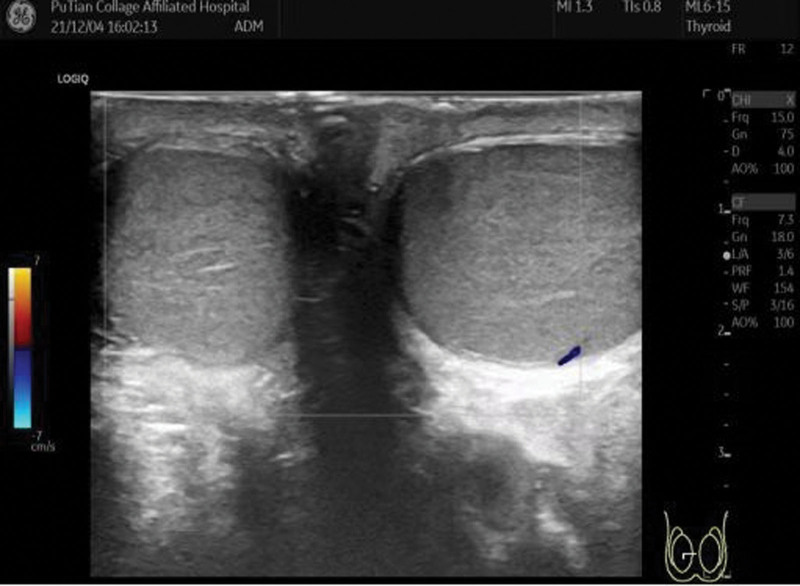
Doppler bilateral comparison.
